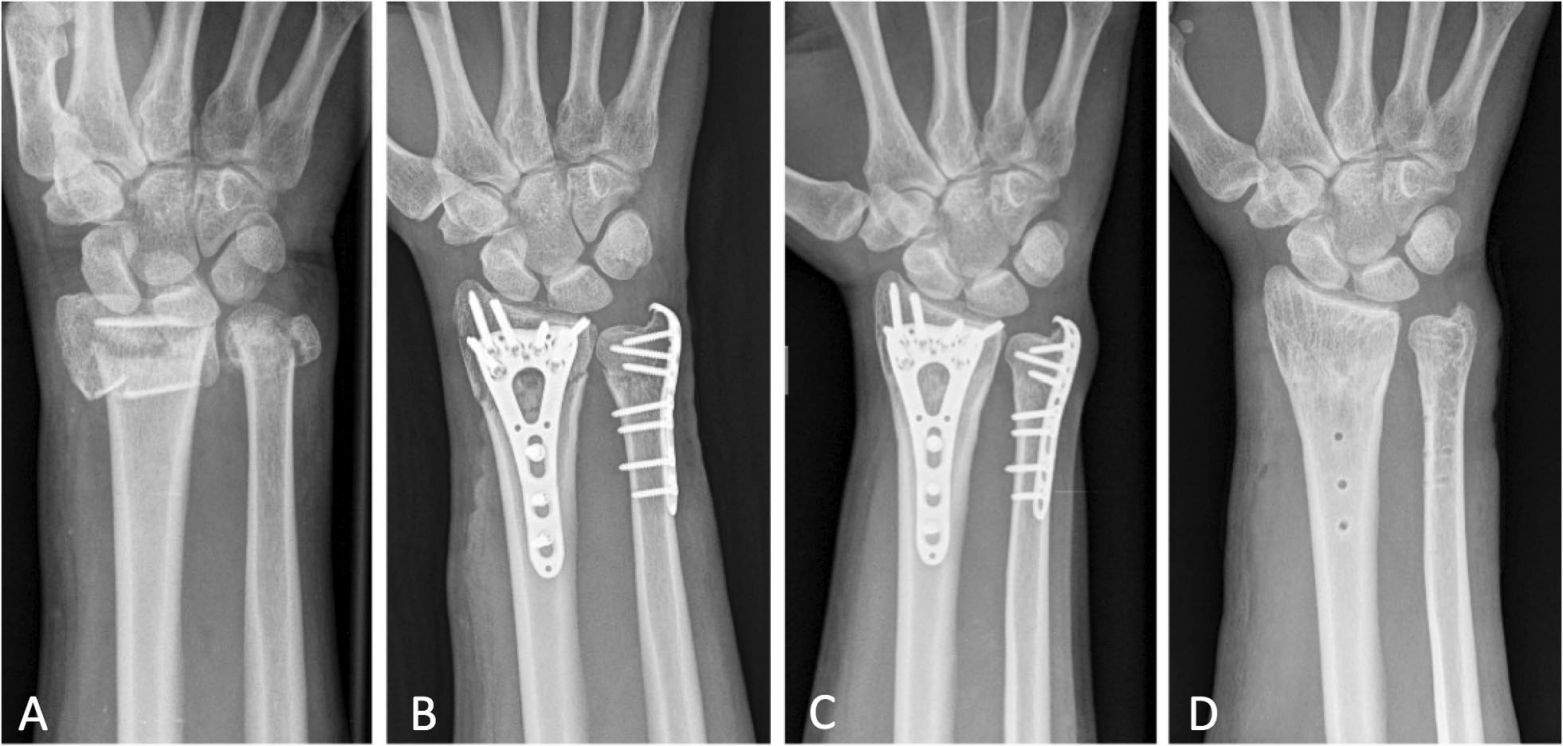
Discomfort after ulna plate osteosynthesis in a young patient. A Preoperative radiograph of a 29-year-old female presenting a Biyani type IV right distal ulna fracture combined with a type C distal radius fracture. B Immediate postoperative radiograph showing right wrist osteosynthesis with ulna hook plate and radius volar plate. C One-year postoperative radiograph showing ulna and radius fracture union. D Radiograph after the removal of implants due to ulna plate discomfort
